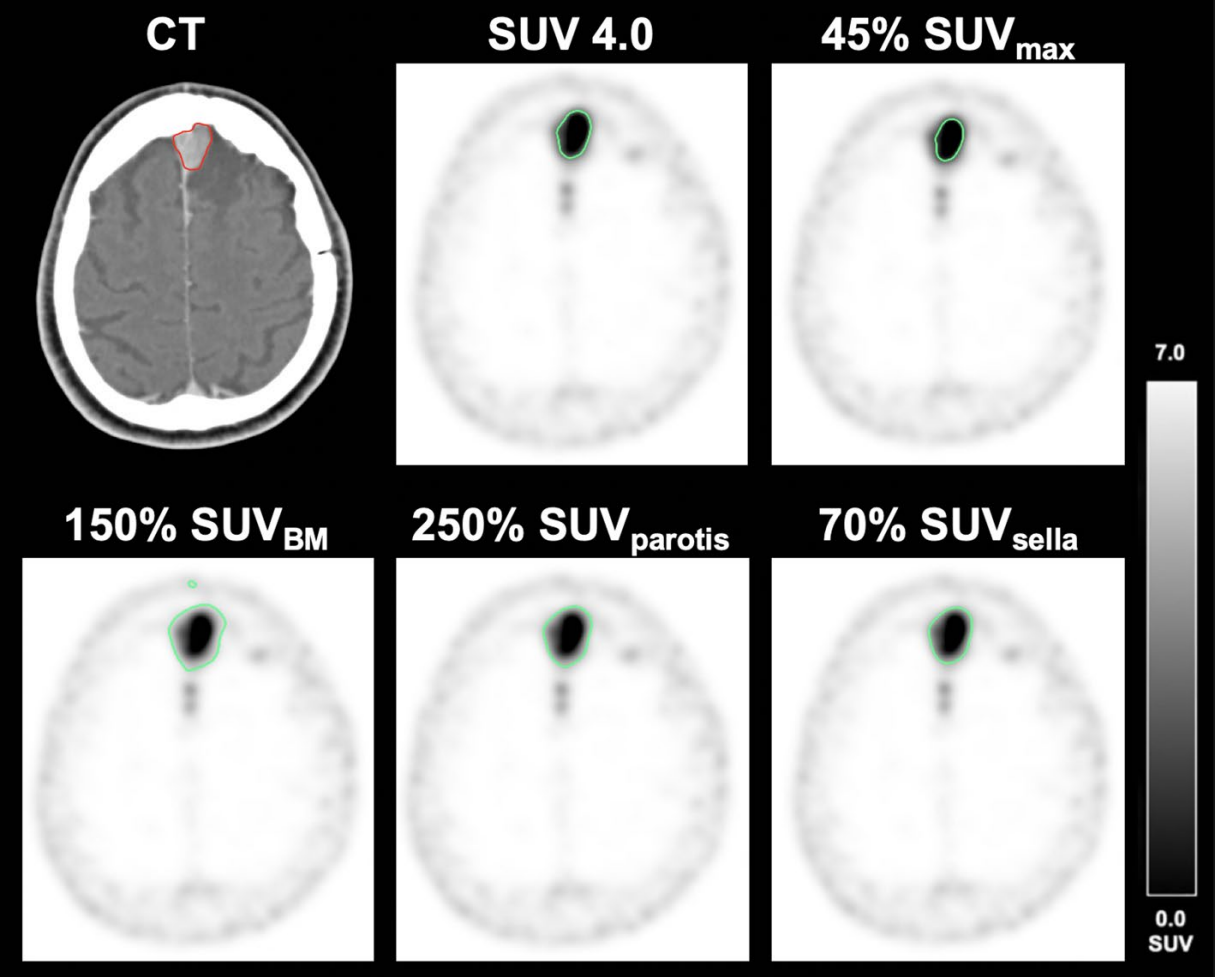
A 57-year-old male patient with meningothelial meningioma, CNS WHO grade 1 at the left falx. The meningioma was delineated applying the best threshold of the respective approach. Volumetric reference standard 4.2 mL; SUV 4.0: 4.2 mL; 45% SUV_max_: 2.2 mL; 150% SUV_BM_: 9.8 mL; 250% SUV_parotis_: 10.2 mL; 70% SUV_sella_: 8.6 mL
